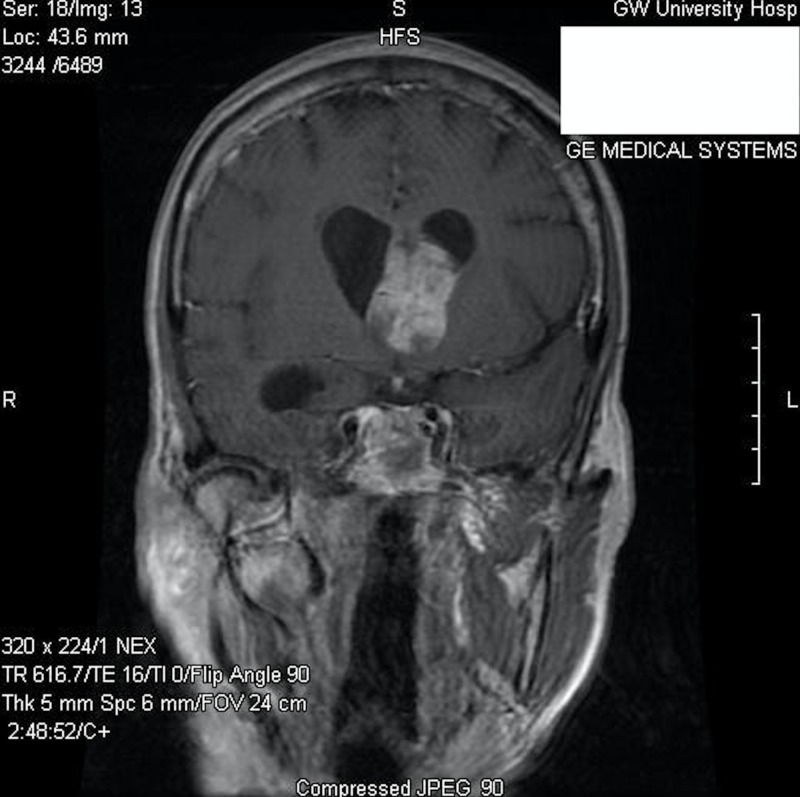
Preoperative coronal post-contrast T1-weighted MR image MR: magnetic resonance
